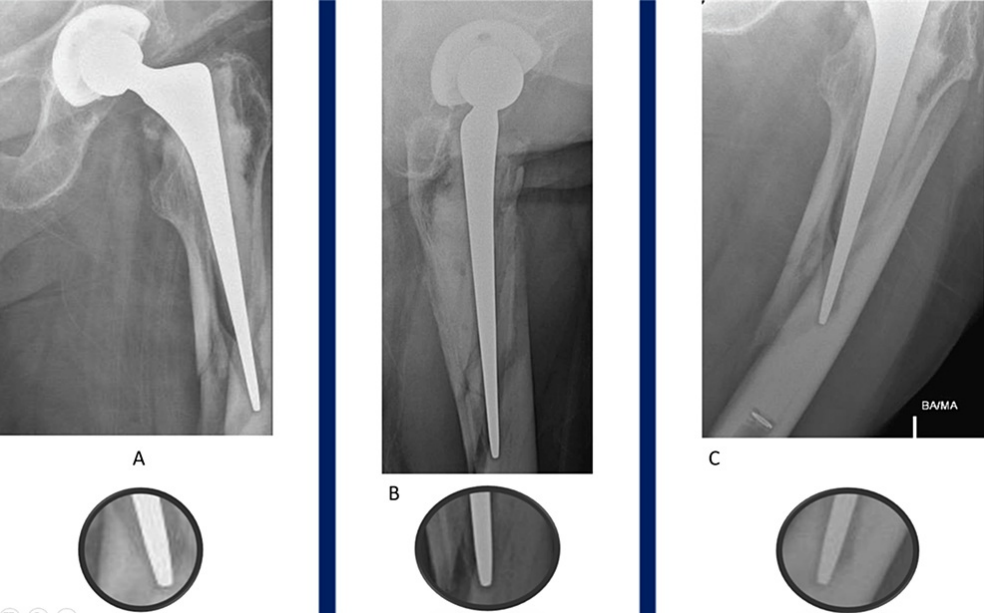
No pre-injury film available AP (A), lateral (B) and oblique (C) radiographs of one patient taken post-injury demonstrating positive ‘SINC’ sign with complete obliteration of the lucency representing the centraliser. No pre-injury film was available.
